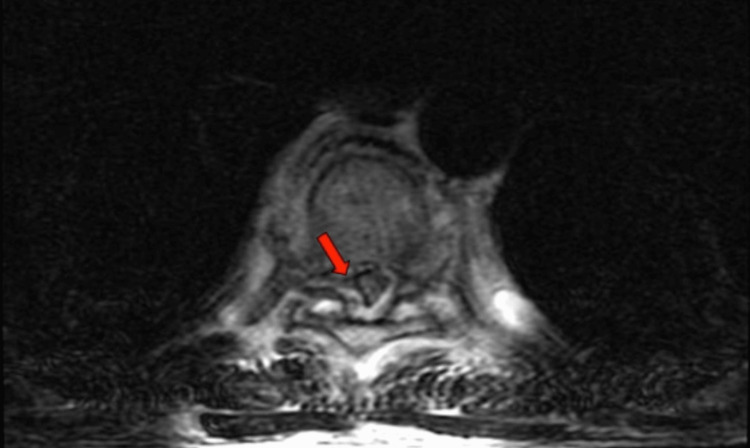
T2-weighted axial view revealing significant compression of the thoracic spinal cord (red arrow).
